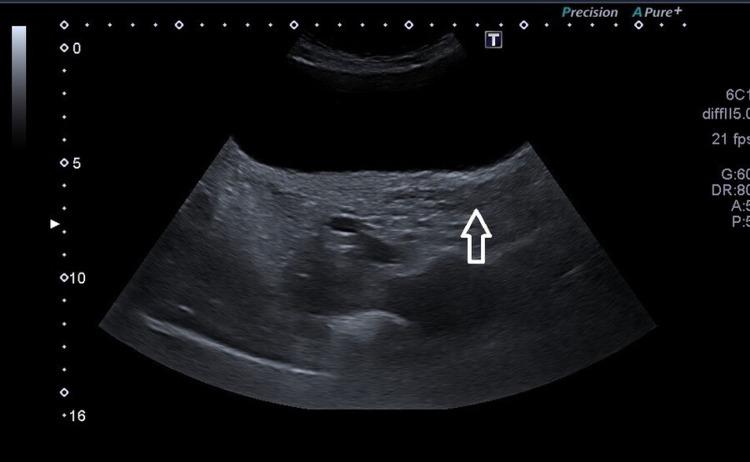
Ultrasound pelvis (arrow showing rudimentary uterus)
